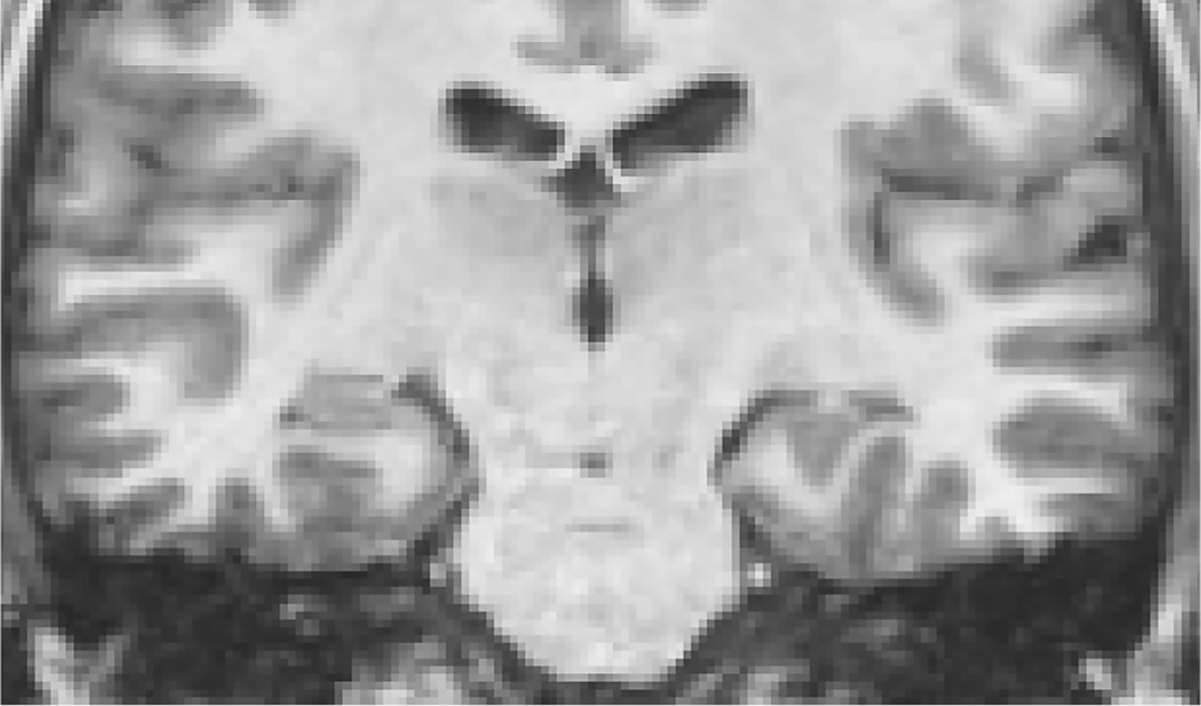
T1 weighted MRI in a coronal view. The left hippocampus (right side in the image) presents an incomplete hippocampal inversion (IHI). The right hippocampus (left side in the image) is an example of a normal or properly inverted hippocampus.
